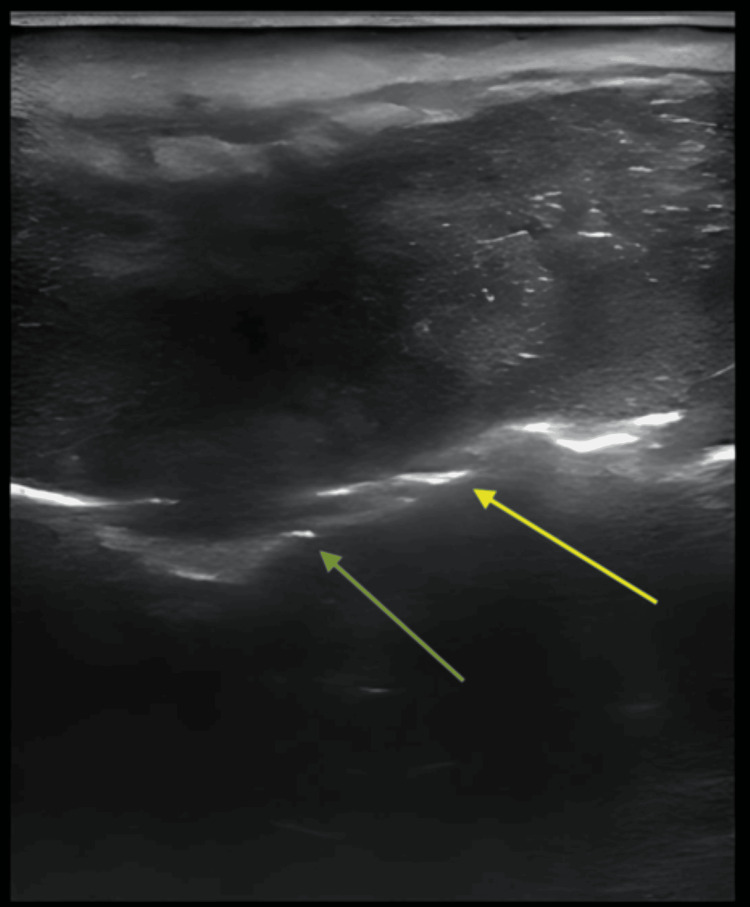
Ultrasound evaluation of oral cavity tumor with cortical bone involvement Ultrasound of the floor of the mouth demonstrates a hypoechoic infiltrative lesion involving the oral mucosa and adjacent soft tissues. The mandibular cortex is identified as a hyperechoic linear structure (yellow arrow), with focal irregularity suggestive of cortical bone invasion (green arrow).
